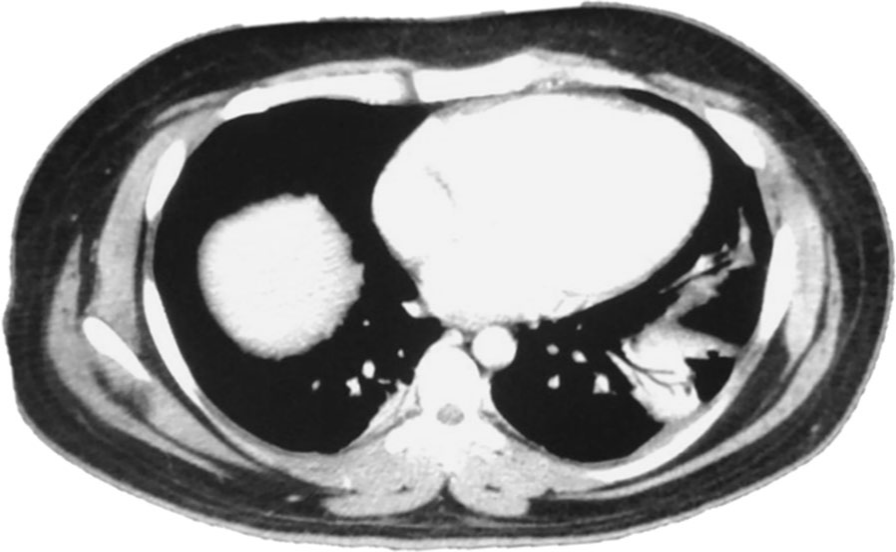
Chest CT showing atelectasis and a left sided pleural effusion
